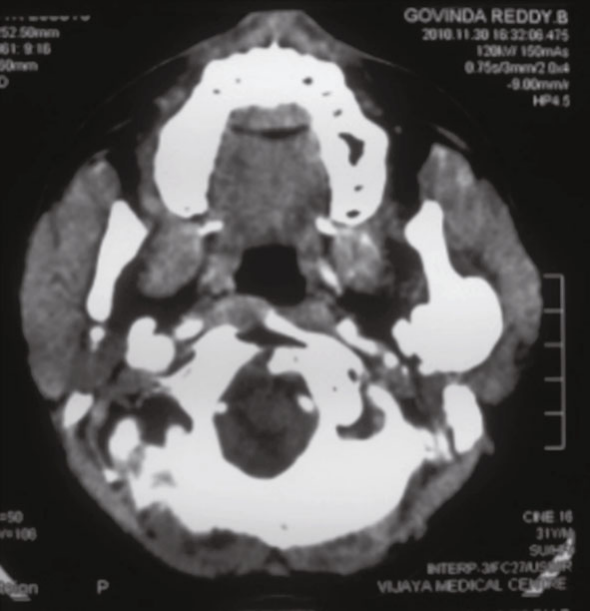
Magnetic resonance imaging scan showed continuity between the medullary cavity of the mandible and the outgrowth. It is isointense on T1 W images and hyperintense on T2 W images with the peripheral hypointense rim on the T1 W and T2 W images; these features are suggestive of the cartilage cap with mineralization.
